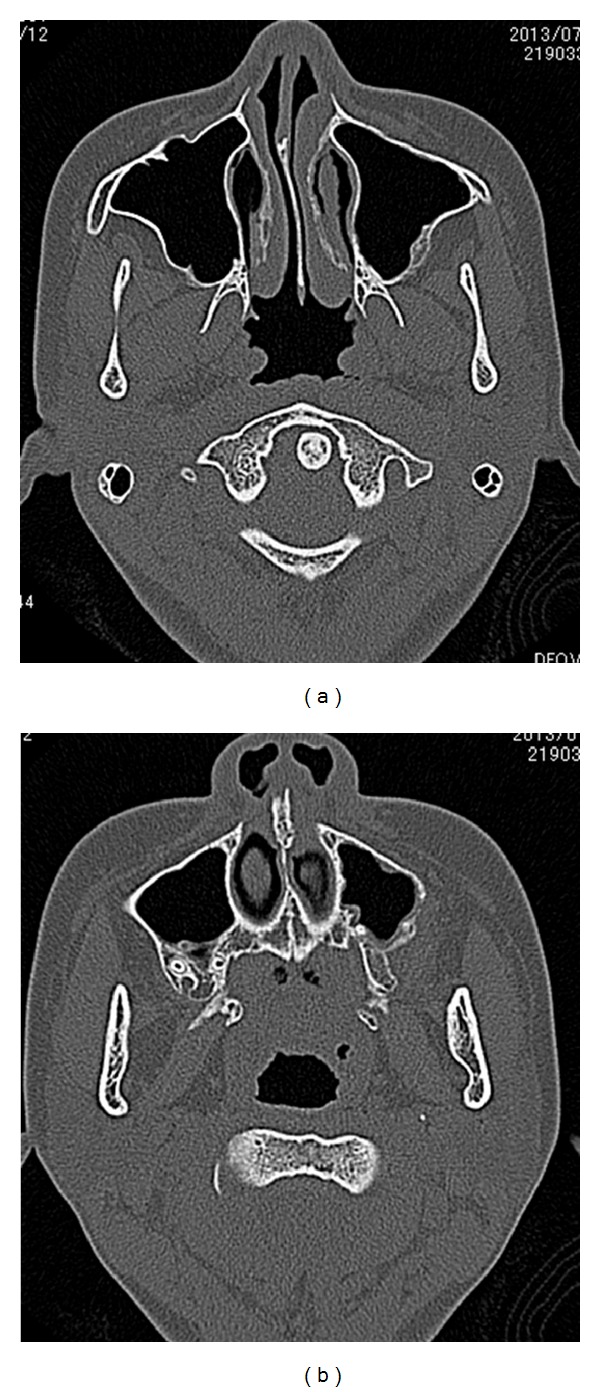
Axial CT view of the lesion.
